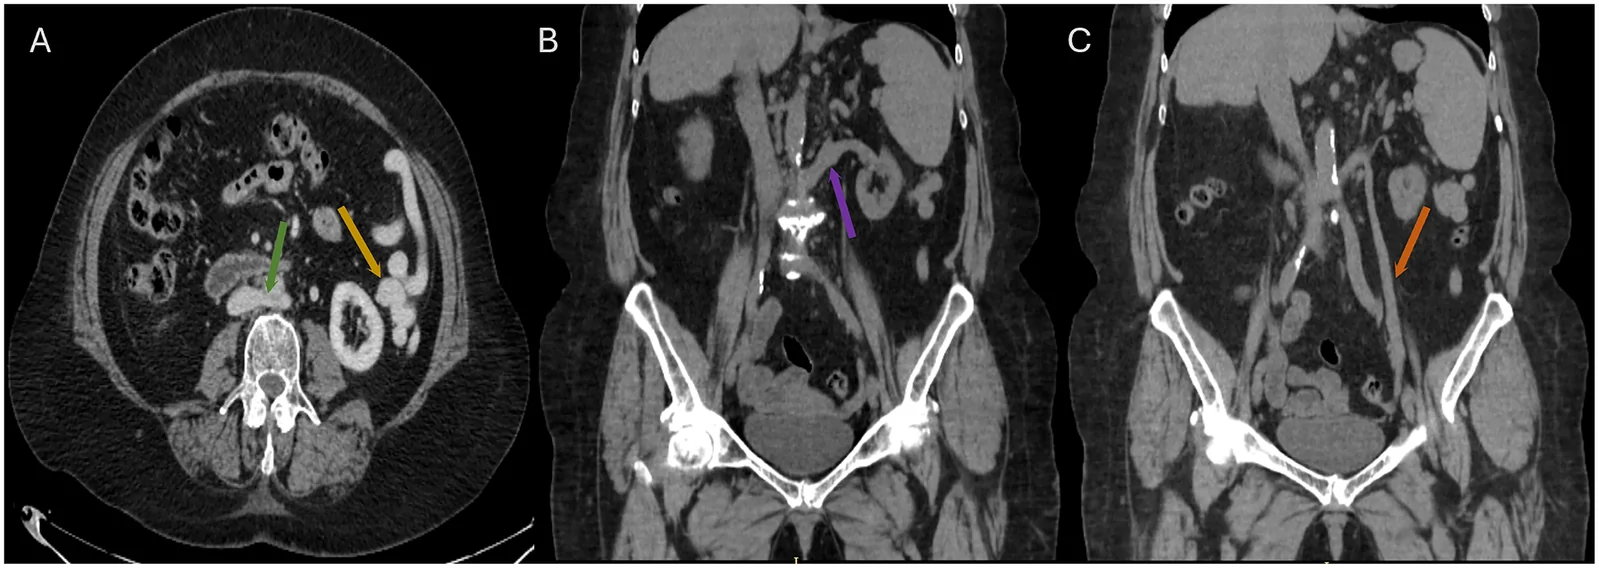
Diagnostic CT findings. (A) Axial venous-phase CT showing (1) marked compression of the retroaortic LRV between the abdominal aorta and vertebral body, with a minimal diameter of approximately 3 mm (green arrow) and (2) collateral venous drainage through splenorenal pathways (yellow arrow). (B) Pre-stenotic dilatation of the LRV measuring approximately 15 mm (purple arrow), yielding a pre-stenotic-to-compressed segment diameter ratio of approximately 5:1. (C) Descending retroperitoneal pararenal collateral drainage toward the left gonadal vein (orange arrow).
